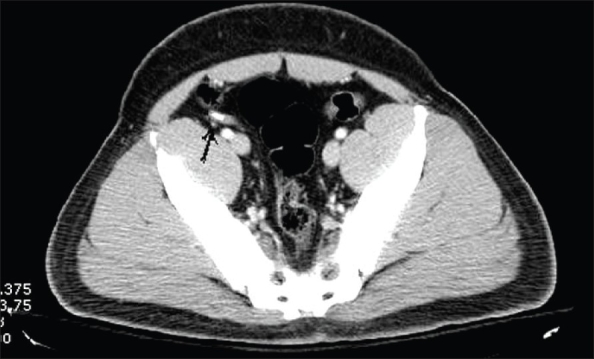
Appendicolith without appendicitis. Contrast-enhanced CT scan shows a nonobstructive appendicolith (arrow) in a normal-caliber appendix without periappendiceal inflammation
